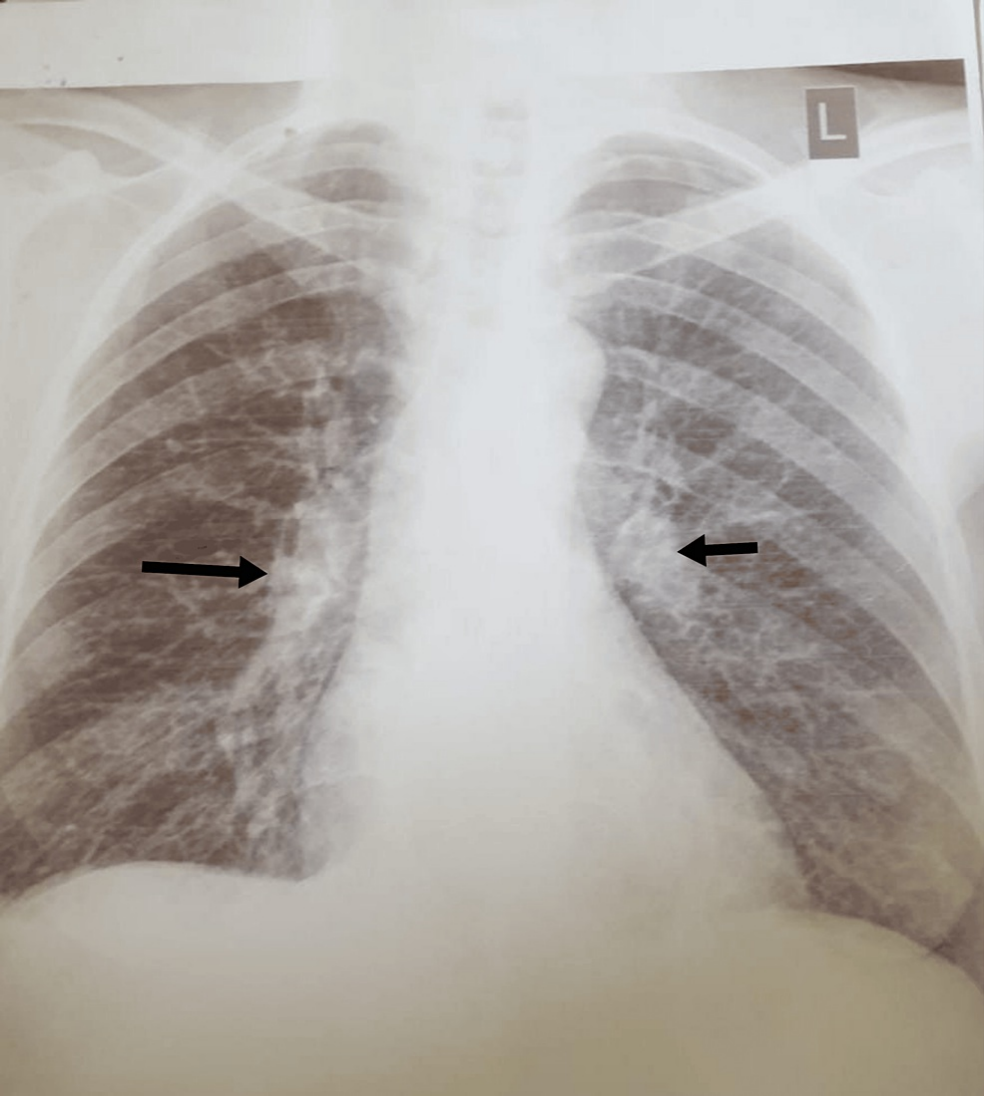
Chest X-ray showing bilateral mediastinal lymphadenopathy.
